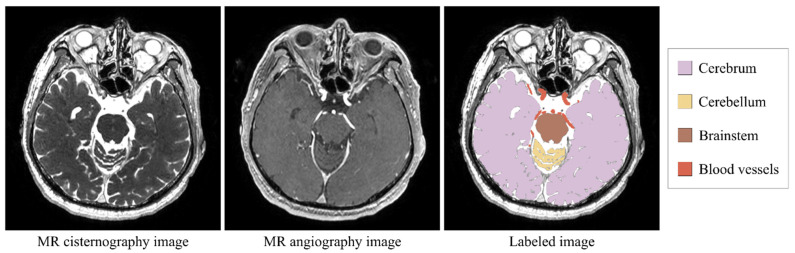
MR images used in this study.
